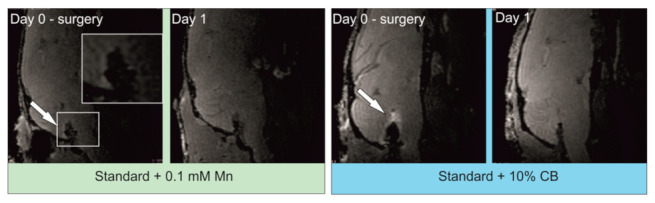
T1-weighted MRI of alginate hydrogels supplemented with 0.1 mM Mn^2+^ or compact manganese alginate particles directly and 24 h after intrathecal transplantation. Arrow indicates a hyperintense signal that corresponds to hydrogel localization. A cropped image of the selected region of interest presents the magnification of the intrathecal area with a hyperintense signal. No signal can be observed after 24 h.
